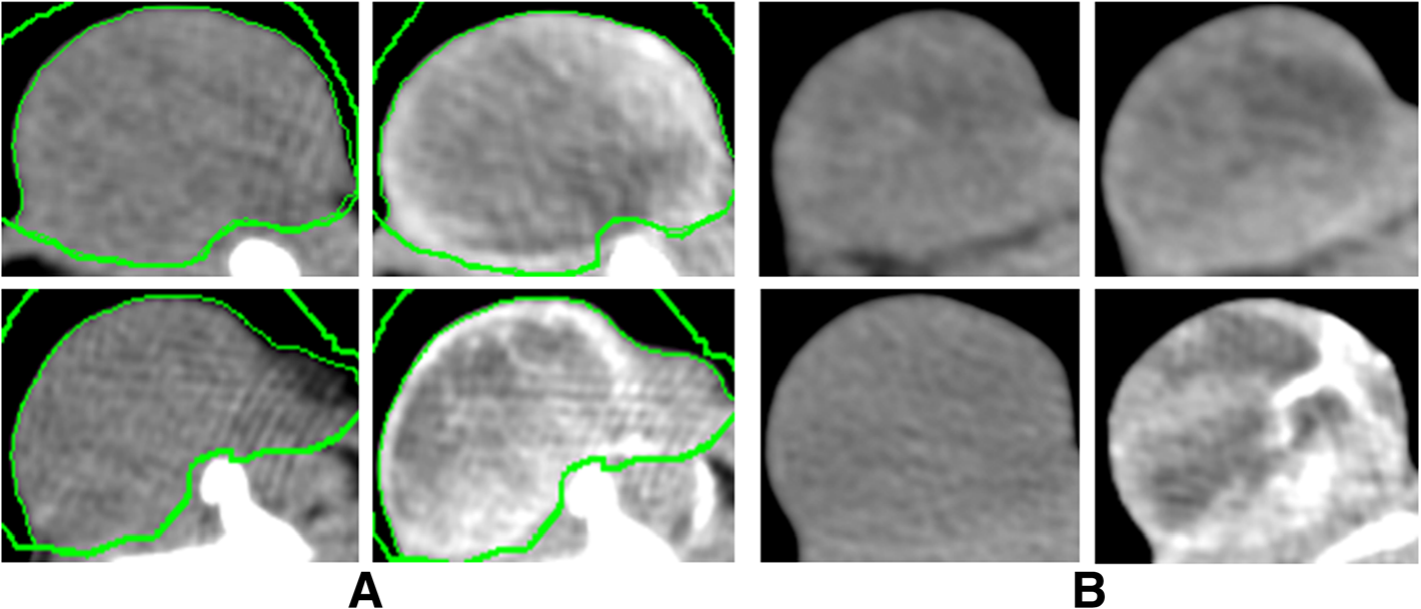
Imaging tumors with CE-CT and native CT. Panel a and b show details of xenograft tumors for cell line A549 and H1299, respectively. The upper and lower rows depict images for non-co-transplanted and co-transplanted tumors, respectively. The left and right columns in a and b depict images from native and contrast-enhanced images. Compare the different degree of contrast enhancement between non-co-transplanted (upper row) and co-transplanted (lower row) tumors. a Upper row: non-co-transplanted tumor with moderate contrast enhancement from native (left column) to contrast-enhanced (right column) image. Lower row: co-transplanted tumor with high contrast enhancement from native (left column) to contrast-enhanced (right column) image. b Upper row: non-co-transplanted tumor with modest contrast enhancement from native (left column) to contrast-enhanced (right column) image. Lower row: co-transplanted tumor with high contrast enhancement from native (left column) to contrast-enhanced (right column) image and vessel within the tumor becoming visible in co-transplanted contrast-enhanced image
